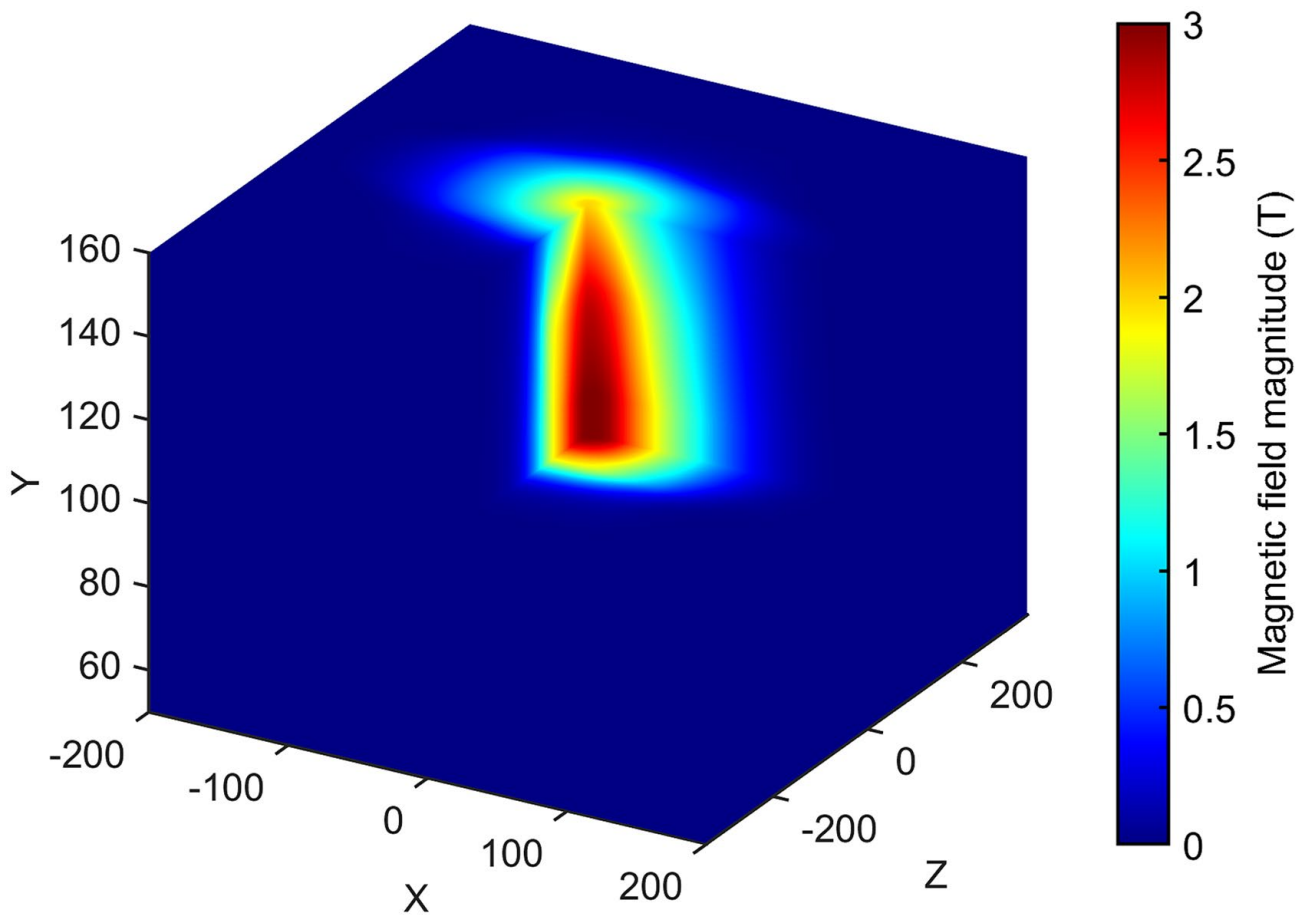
3D map of the magnetic field magnitude |B| in the entire MRI room (isocentre is at *x* = 0, *z* = 0, *y* = 105 cm). The external part has been removed to show the isocentre zone.
